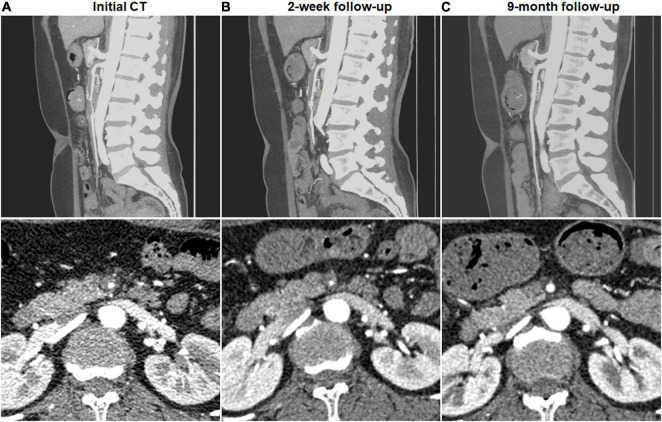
Complete remodeling of type IV spontaneous isolated superior mesenteric artery dissection on follow-up computed tomography (CT) angiography. (A) Initial CT angiography showed the completely thrombosed false lumen in a 44-year-old woman. (B) Two weeks later, false lumen thrombus became thickened, and stenosis of true lumen was aggravated. (C) Nine months later, false lumen thrombus was completely resolved, and type IV dissection achieved complete remodeling.
